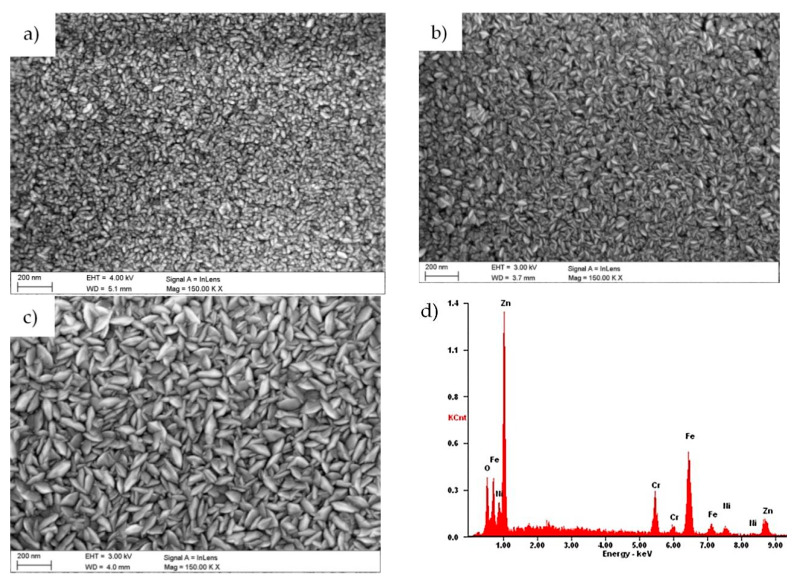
Surface morphology of the sample with the ZnO coating deposited in (a) 500; (b) 1000; and (c) 1500 ALD cycles; (d) X-ray energy dispersive plot of the area shown in Figure 2c.
